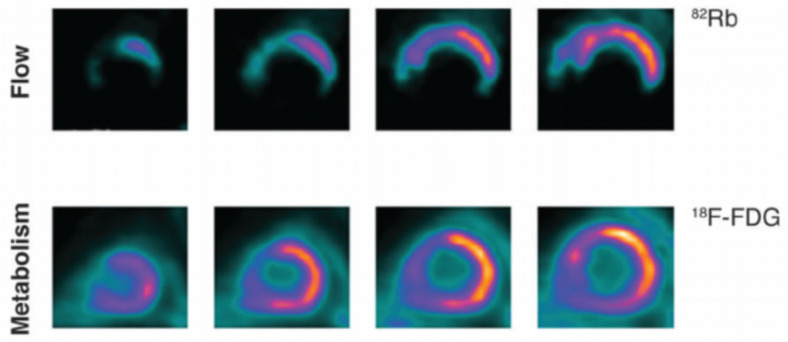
FDG-PET imaging demonstrating perfusion–metabolism mismatch—reduced perfusion (top row) with preserved glucose uptake (bottom row)—consistent with viable but hibernating myocardium. Adapted from Osterholt et al., “Targeted Metabolic Imaging to Improve the Management of Heart Disease,” under open-access terms [25].
